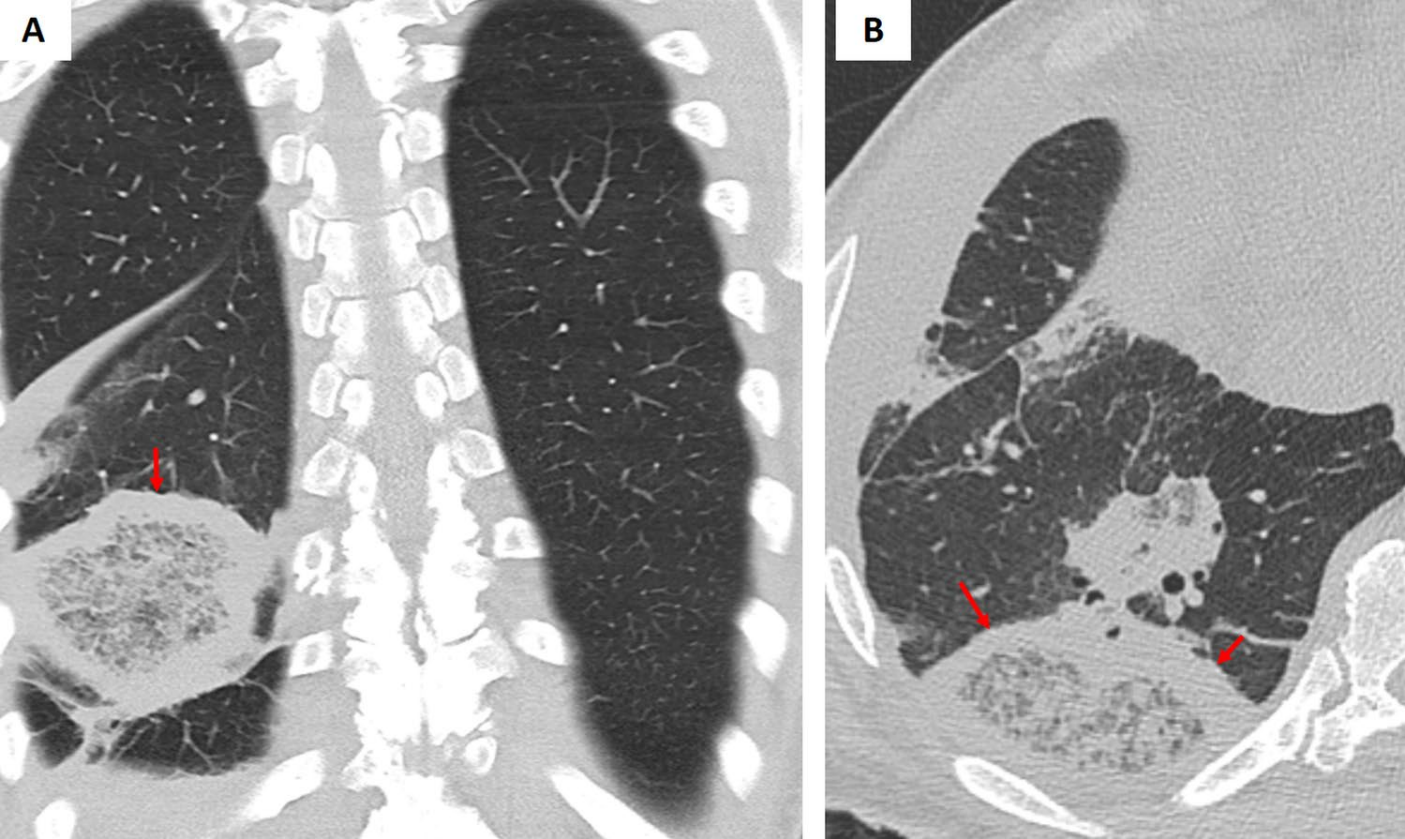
A Coronal and (B) axial chest CT images of a patient with acute myeloid leukemia (AML) demonstrate a right lower lobe peripheral pulmonary opacity with a bird’s nest sign (arrows). This finding represents a reverse halo sign characterized by irregular and intersecting lines or stranding within an area of ground-glass opacity, indicative of invasive fungal infection. The patient was subsequently diagnosed with mucormycosis
